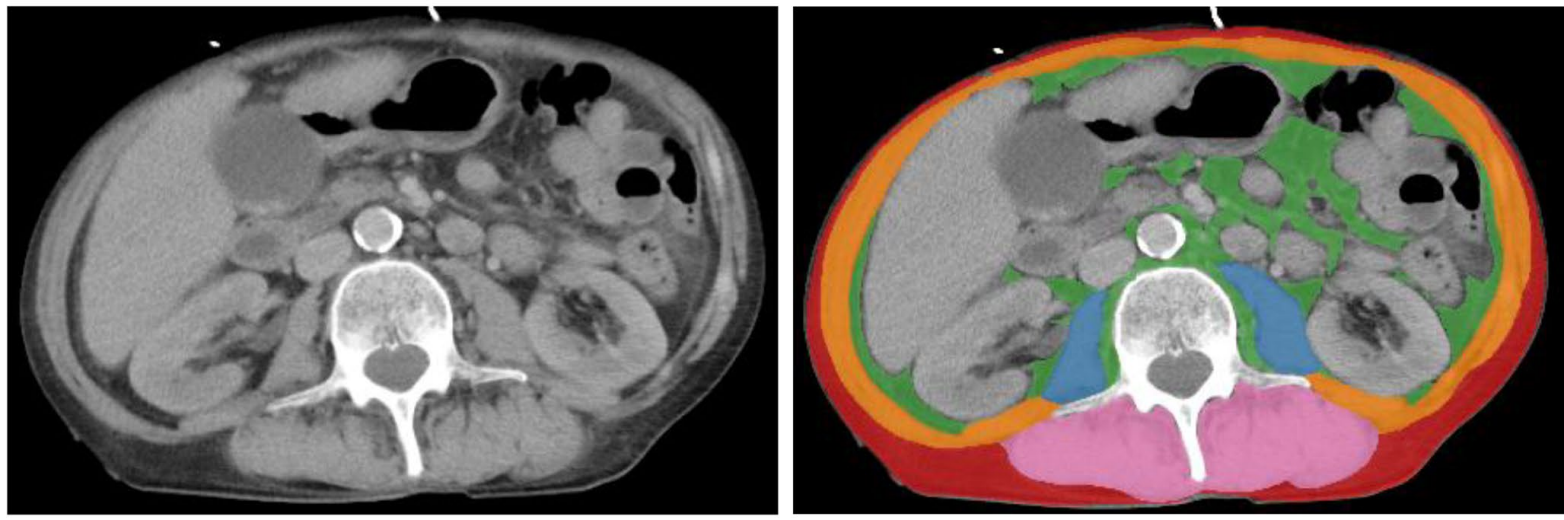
Example of an area analysis of CT-BCPs on the L3 level of a CT scan. Measurement of visceral adipose tissue area: 59.1 cm^2^ (green), subcutaneous adipose tissue area: 52.9 cm^2^ (red), psoas muscle area: 17.7 cm^2^ (blue), abdominal muscle area: 61.6 cm^2^ (orange), long spine muscle area: 51.5 cm^2^ (pink)
